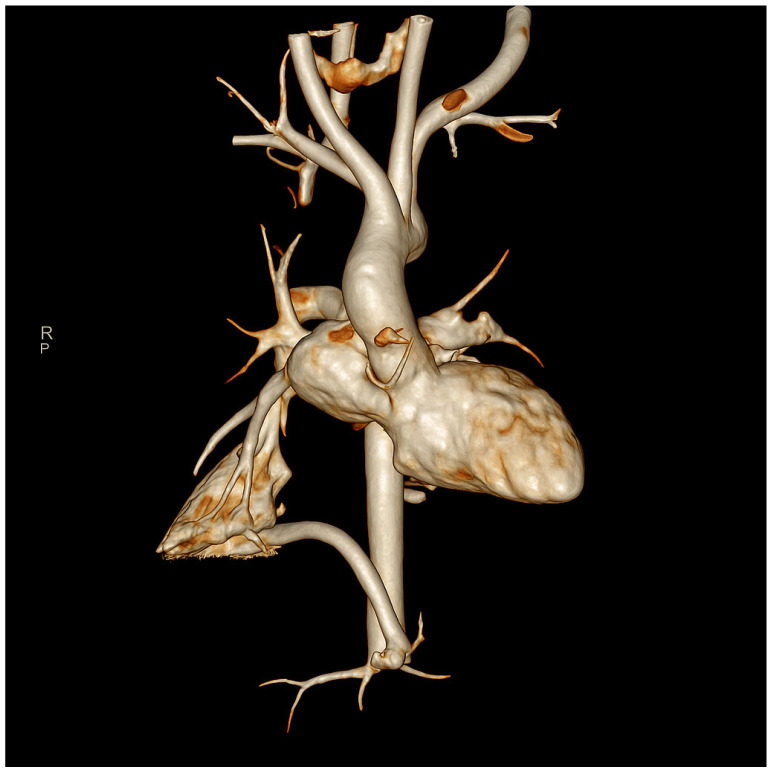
Three-dimensional volume-rendered reconstruction from contrast-enhanced CT angiography showing an anomalous systemic arterial supply to the right lower lobe arising from the celiac trunk, consistent with pulmonary pseudosequestration.
